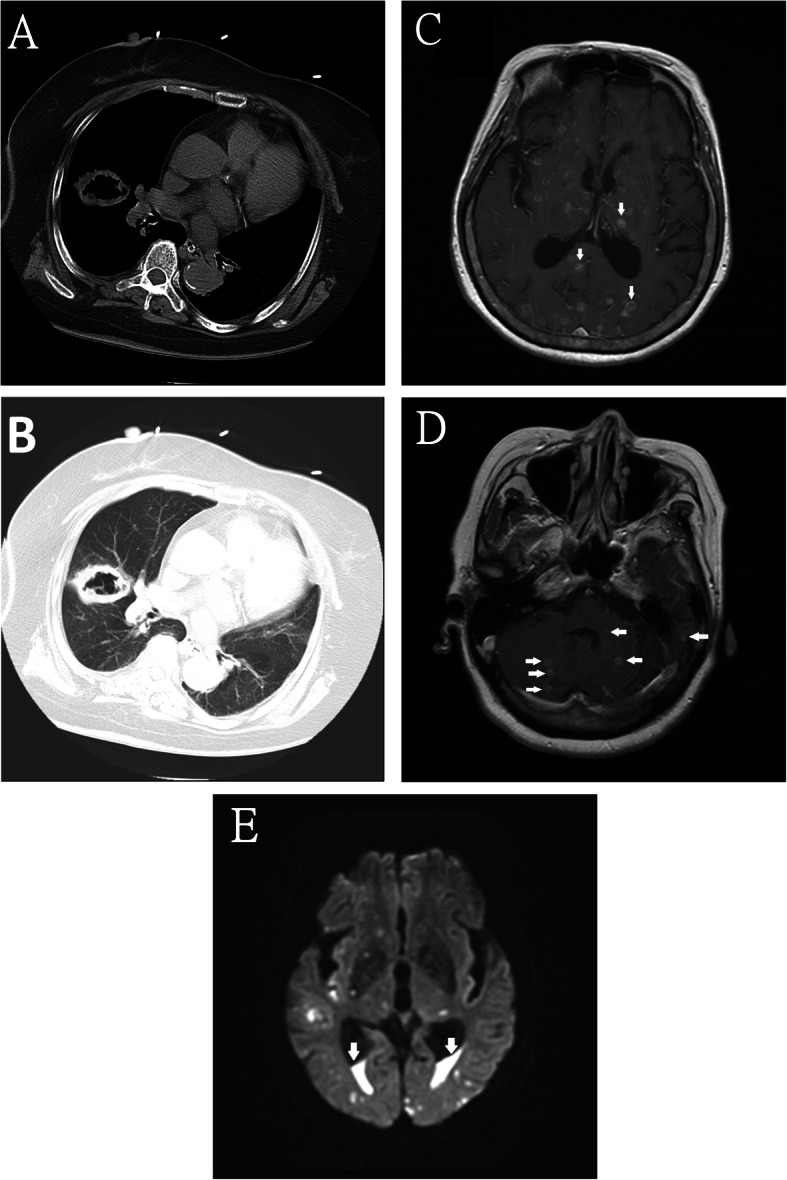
The chest computed tomography and brain magnetic resonance imaging. The chest computed tomography showed a 5.2 cm cavity containing some small ball-like lesions and slight pericardial effusion (Fig. 2a and b). The brain magnetic resonance imaging showed multiple contrast-enhanced cystic lesions with surrounding brain edema and eccentrically hyperdense lesions within the cysts in the cerebrum, cerebellum, and the brain stem (Fig. 2c, d) and abnormal fluid accumulation in the dependent portion of both lateral ventricles (Fig. 2e)
